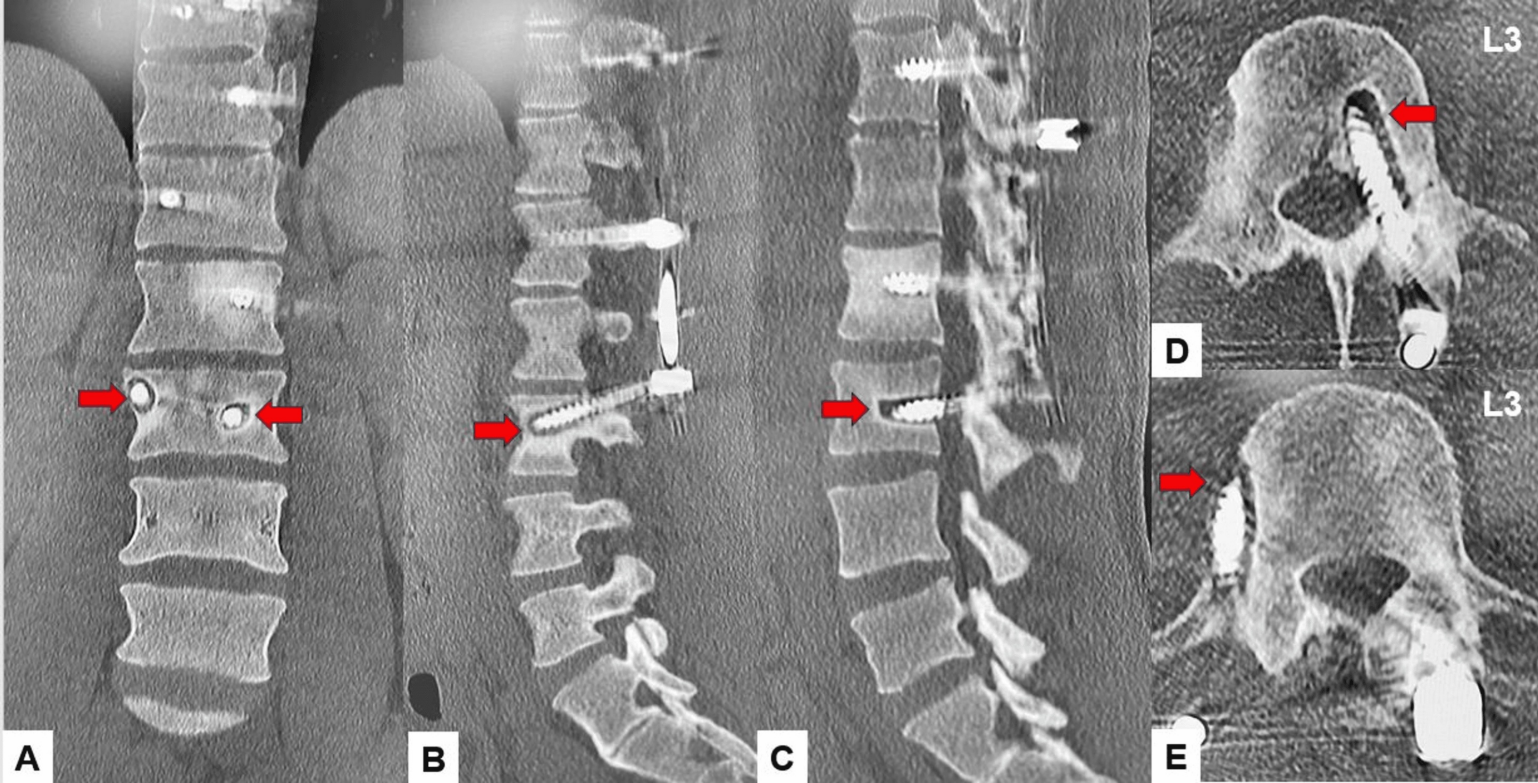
Five-year follow-up images A: Coronal lumbar reconstruction CT, B: Right parasagittal lumbar reconstruction CT, C: Left parasagittal lumbar reconstruction CT, and D and E: Axial CT at L3. Red arrows indicate screw loosening.
